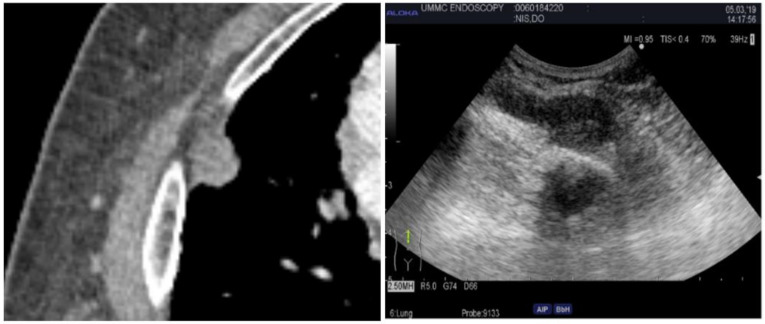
Computed tomography of the chest (Left) and corresponding percutaneous ultrasound (Right) of the nodule. The ultrasound image demonstrates ribs, pleura, and the nodule.
